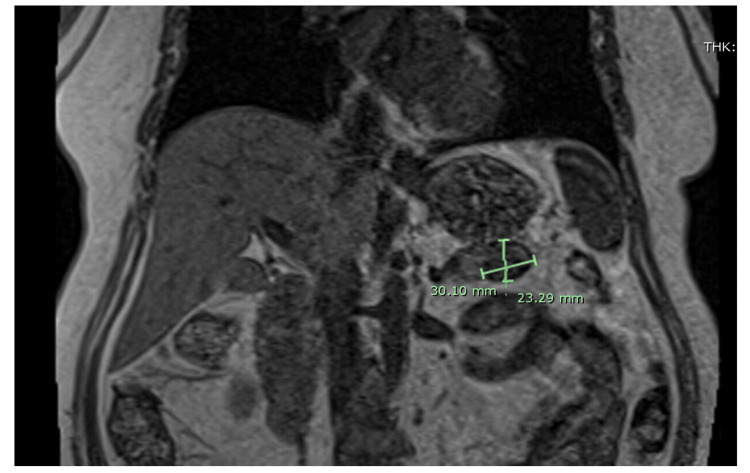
Magnetic resonance imaging of the abdomen and pelvis Pancreatic tail mass without significant enlargement since 2018 and demonstrating signal characteristics similar to the spleen is suggestive of an intrapancreatic splenule. No main pancreatic ductal dilatation. No suspicious lymphadenopathy.
